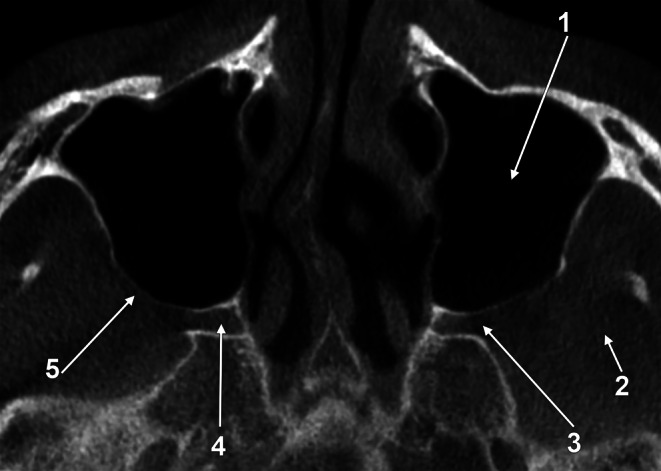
Axial CBCT slice through the maxillary tuberosities. (1) maxillary sinus; (2) infratemporal fossa; (3) pterygomaxillary fissure; (4) pterygopalatine fossa; (5) maxillary tuberosity on the thin posterior antral wall
